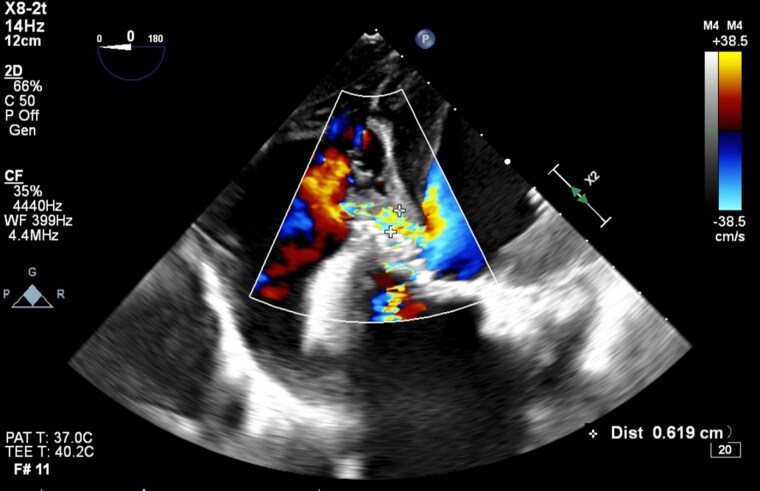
Intraoperative transoesophageal echocardiogram image showing flow across ventricular septal defect with a diameter measured at 0.619 cm. Jet location indicated by white crosses.
